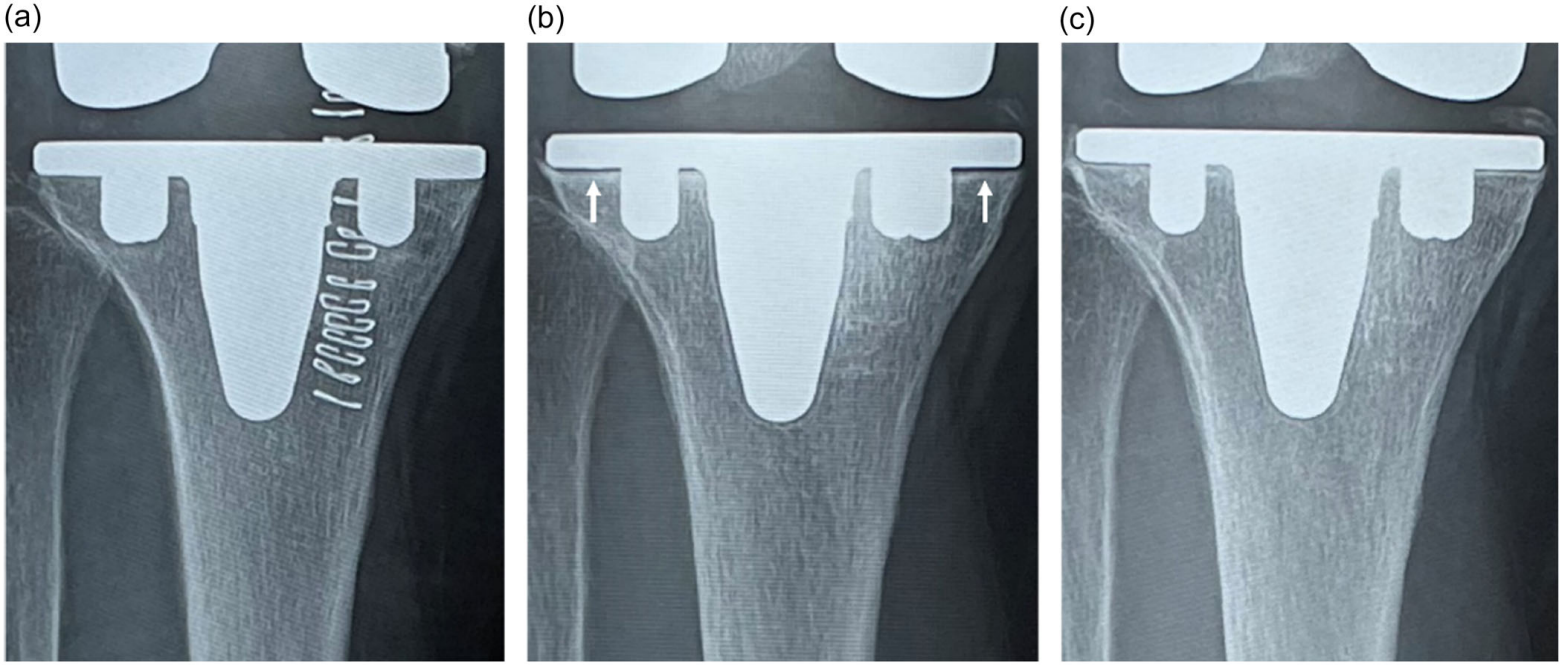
(a) AP radiographs of a patient who underwent POROCOAT TKA at 1 week postoperatively. (b) AP radiographs of a patient who underwent POROCOAT TKA at 6 months postoperatively. RLLs appeared in Zones 1 and 2 (arrow). (c) AP radiographs of a patient who underwent POROCOAT TKA at 1 year postoperatively. RLLs disappeared. AP, anterior–posterior view; RLLs, radiolucent lines; TKA, total knee arthroplasty.
